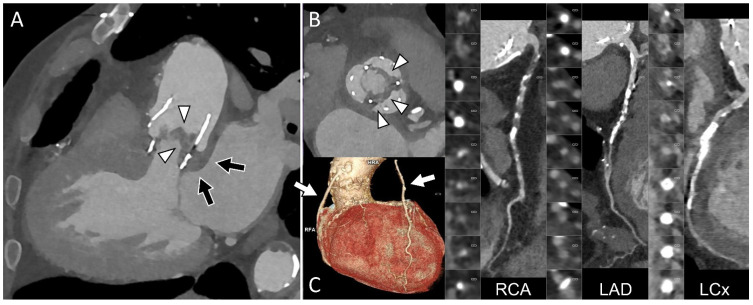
Comprehensive cardiac CT assessment in prosthetic valve endocarditis involving a Perceval sutureless valve. Echocardiography was diagnostic for valvular vegetations, but the presence and extent of perivalvular involvement remained uncertain. Cardiac CT was therefore performed to evaluate periprosthetic complications and to assess coronary anatomy prior to surgery. Three-chamber (A) and aortic plane (B) views confirm leaflet vegetations (arrowheads) associated with extensive periprosthetic soft-tissue thickening extending to the mitral–aortic fibrosa (black arrows), consistent with a perivalvular abscess ((A), black arrows). CT coronary angiography demonstrated severe and diffuse coronary artery disease but patency of two coronary bypass grafts ((C), arrows), neither of which was in close proximity to the predicted re-sternotomy plane.
